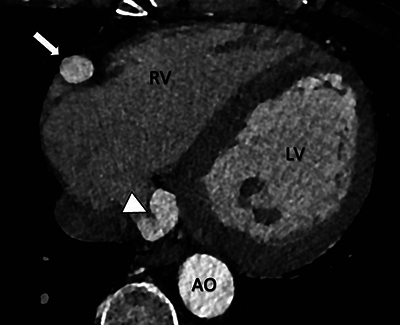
Cardiac CT-angiography reveals a coronary artery-to-coronary sinus fistula (white arrowhead). Note the dilation of the right coronary artery (white arrow). Axial view.
